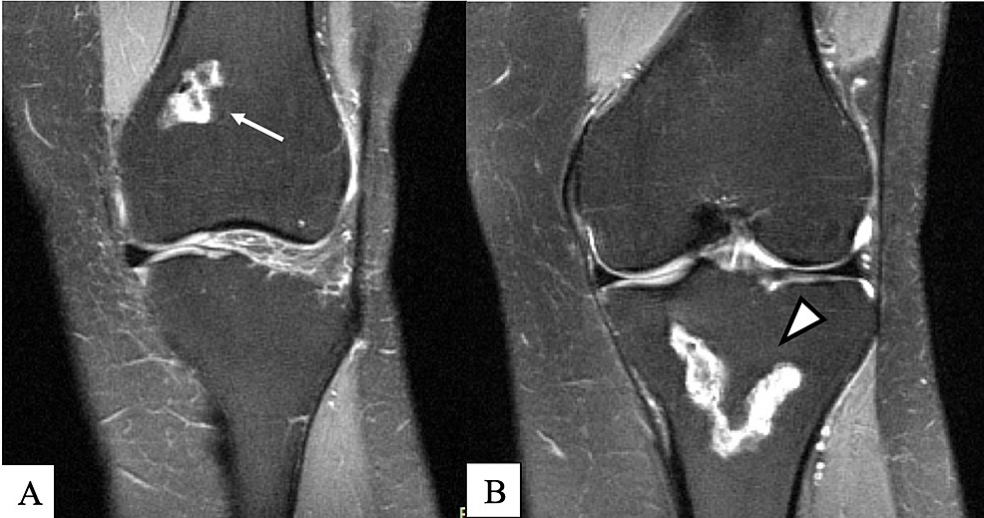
MRI dated June 2019 Proton density fat saturation without contrast; coronal view demonstrates bone infarct of the distal femoral metaphysis extending to the proximal aspect of the medial femoral condyle (arrow) (A) and bone infarct of the proximal tibial metaphysis with extension into the tibial plateau and periosteal reaction along the tibial metaphysis (arrowhead) (B) MRI: magnetic resonance imaging
